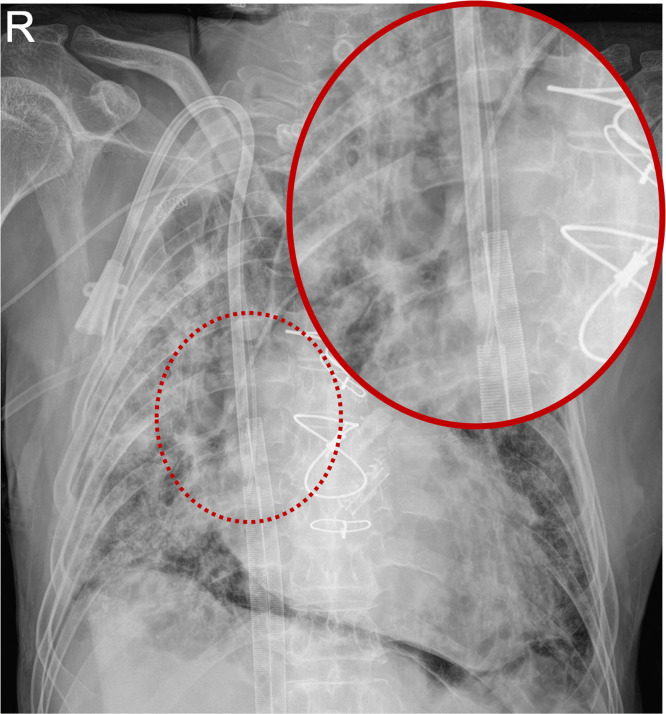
Bedside PICC placement in a complex clinical environment with multiple concomitant intravascular devices. Representative chest radiograph showing the coexistence of a same-side Permcath, a contralateral PICC, and two femoral extracorporeal membrane oxygenation (ECMO) sheaths. Despite the high clinical complexity and potential for device interference, the catheter tip is accurately localized at the target zone using the pre-procedural length prediction formula.
